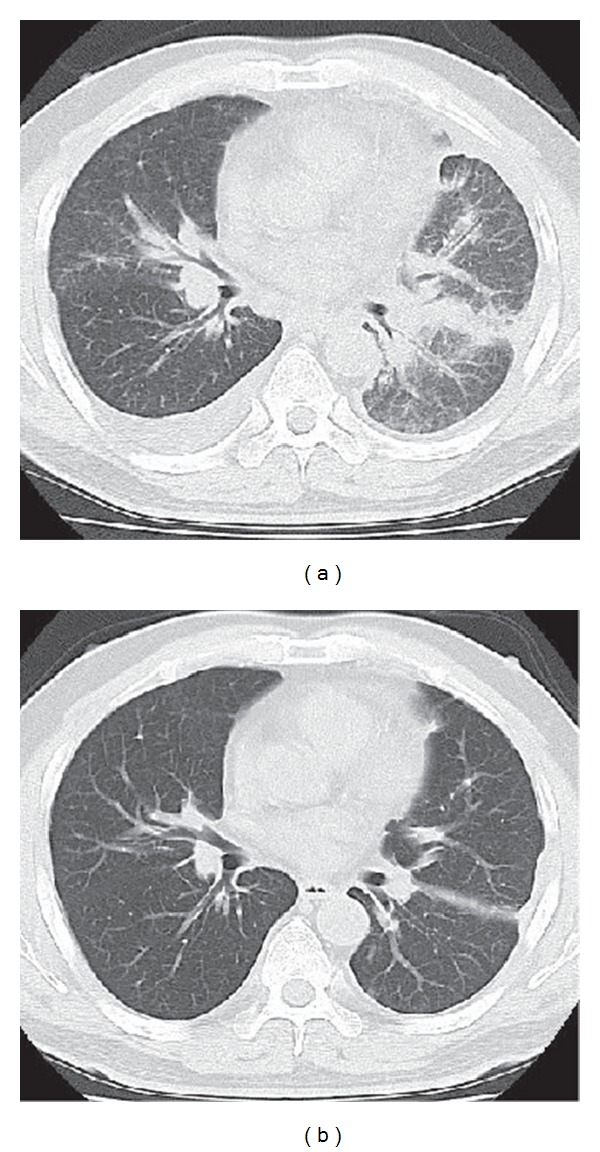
Chest CT of case 1 before chemotherapy demonstrating diffuse pleural dissemination of the tumor and irregular thickening of the bronchovascular bundle, indicating carcinomatous lymphangitis, and right pleural effusion (a). CT after two cycles of chemotherapy showing significant response (b).
